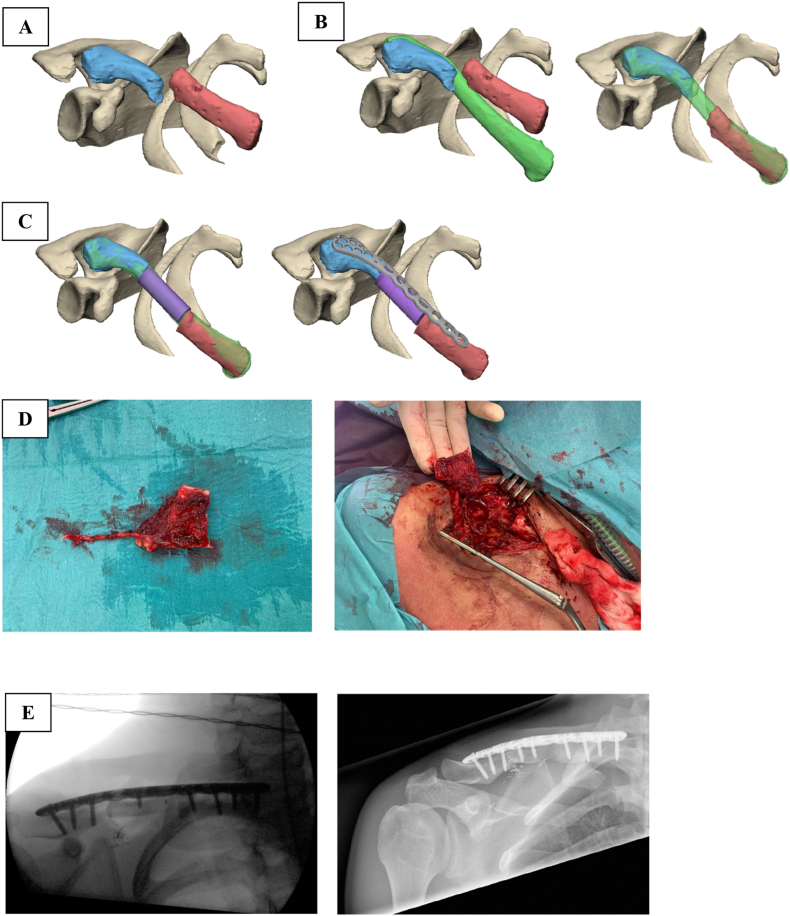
Clavicula reconstruction A 20-year-old female patient underwent vascularized bone reconstruction due to persistent malunion of the right clavicle. (A) Three-dimensional (3D) reconstruction of the affected clavicula. (B) Contralateral clavicula superimposed over the affected side and virtually repositioned. (C) Osteotomy planes, scapular tip graft and osteosynthesis plate position virtually determined. (D) intraoperative photographs of the scapular tip graft before (left) and after (right) reconstruction. (E) Radiographs before (left) and 6 months after reconstruction (right).
